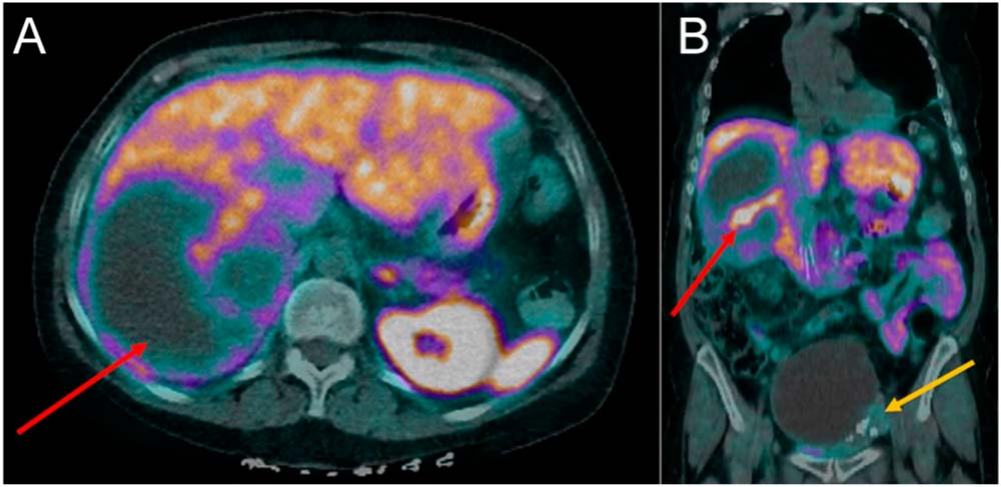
^68^Gallium-DOTATATE PET/CT. (A) Axial view of right adrenal mass (red arrow) demonstrating areas of low uptake due to a cystic, necrotic core. (B) Coronal view of both right adrenal mass (red arrow) and pelvic mass with low GaTate avidity (yellow arrow).
